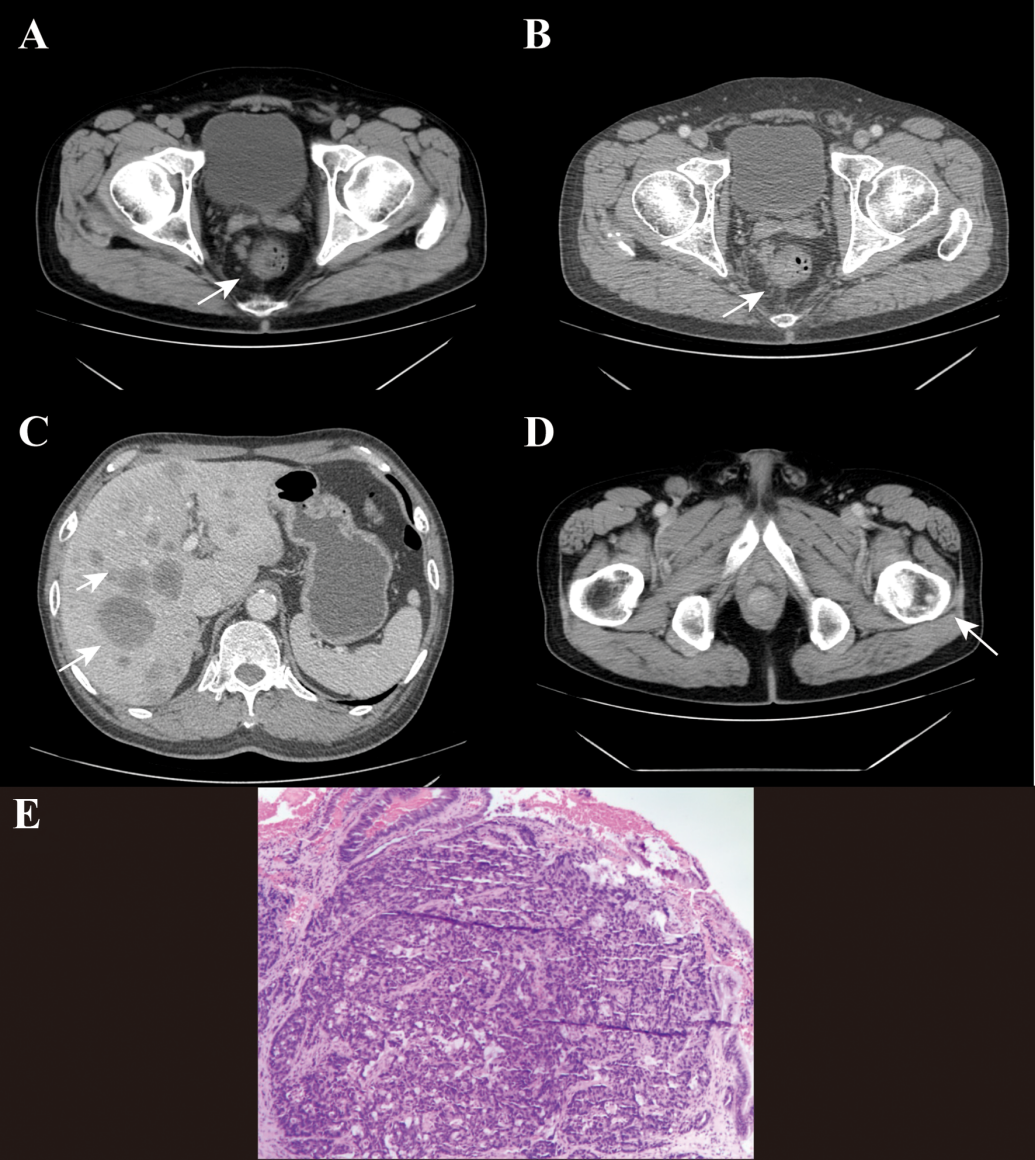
The plain and enhanced computed tomography (CT) scan images of the patient’s abdomen at admission and the pathological biopsy under colonoscopy of the patient. (A) Abdominal CT plain scan shows thickening of the intestinal wall at the terminal rectum. (B) Enhanced abdominal scan of the patient shows significant enhancement of the rectal wall, multiple adjacent lymph nodes and slightly increased density of the mesentery. (C) Multiple low-density, nearly round shadows with indistinct margins are visible in the liver. (D) A nodular slightly high-density shadow is visible on the left femur, with a long diameter of approximately 0.9 cm. (E) The patient’s pathological biopsy showed adenocarcinoma.
